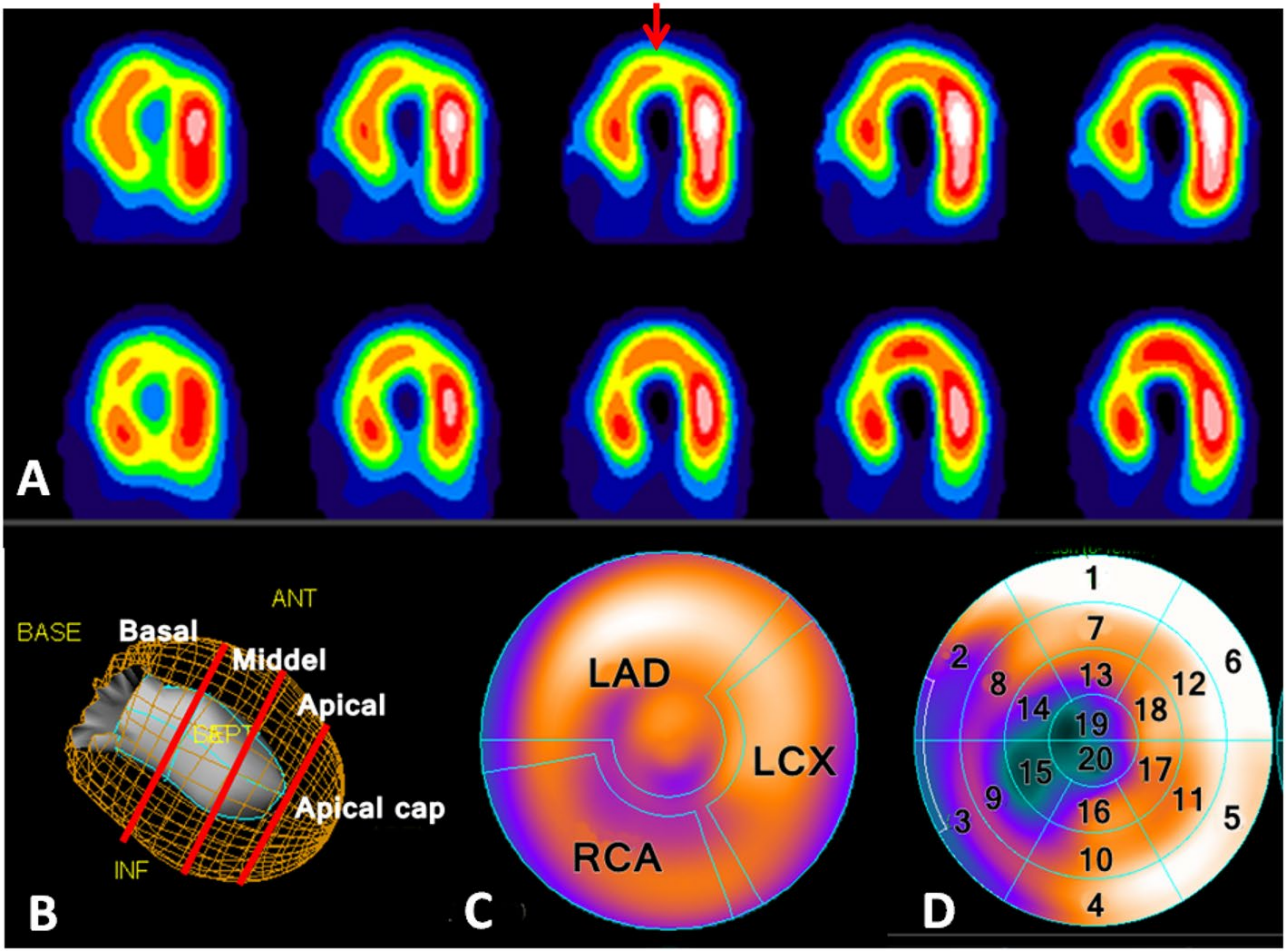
Demonstrations of myocardial perfusion analytic display of the study. (A) MPI SPECT multislice display of conventional qualitative interpretation, under post-stress (upper panel) and redistribution (lower panel) in the horizontal axis, respectively. The arrow shows the stress-induced perfusion defect with revere at apex. (B) Four rings display. LV myocardium was divided into four rings, namely basal, middle, apical, and apical cap. (C) Three territories display based on major coronary arteries supply. (D) Twenty segments display according to AHA’s 20-segment model. *MPI* myocardial perfusion imaging. *SPECT* single photon emission computed tomography. *LV* left ventricle. *AHA* American heart association.
